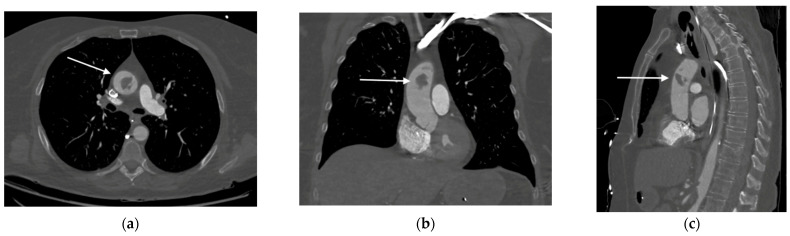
Contrast-enhanced chest CT revealing the presence of a mass (as indicated by the arrows) in the ascending aorta: (a) axial; (b) coronal; and (c) sagittal views.
